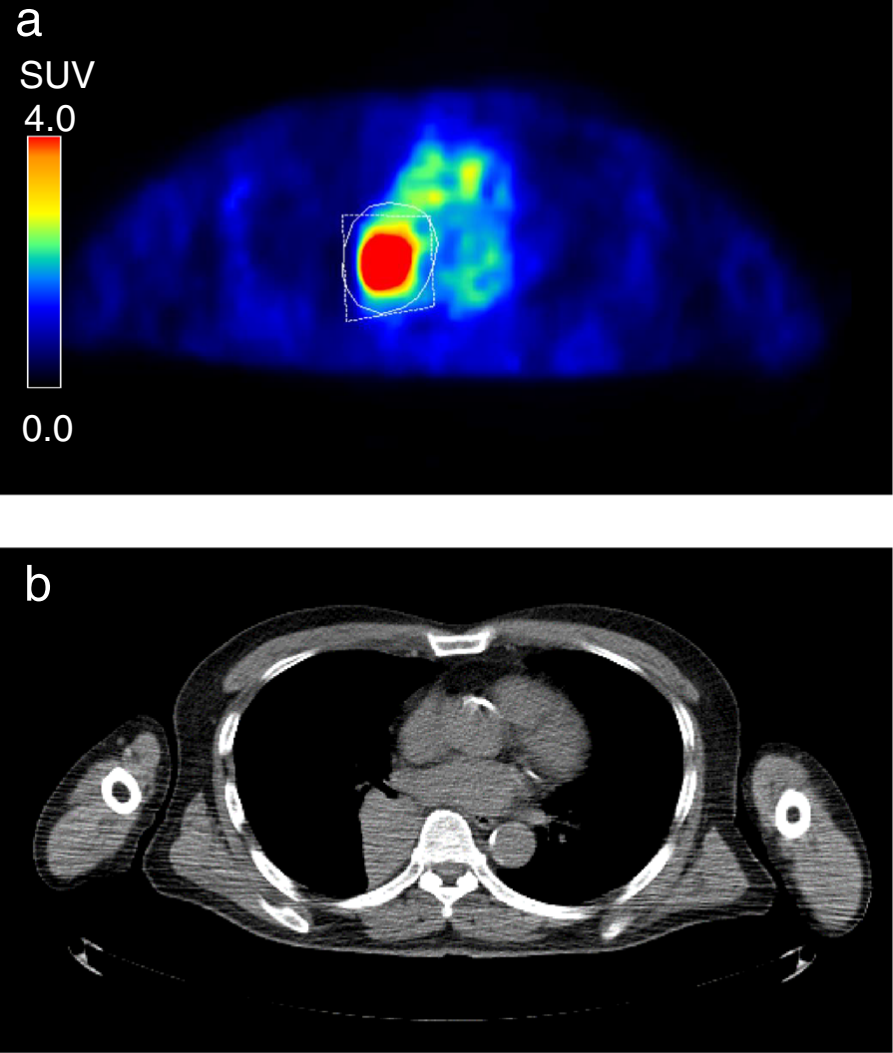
a Regions of interest defined by Operator-1 (solid line) and Operator-2 (dashed line). b Corresponding CT slice
